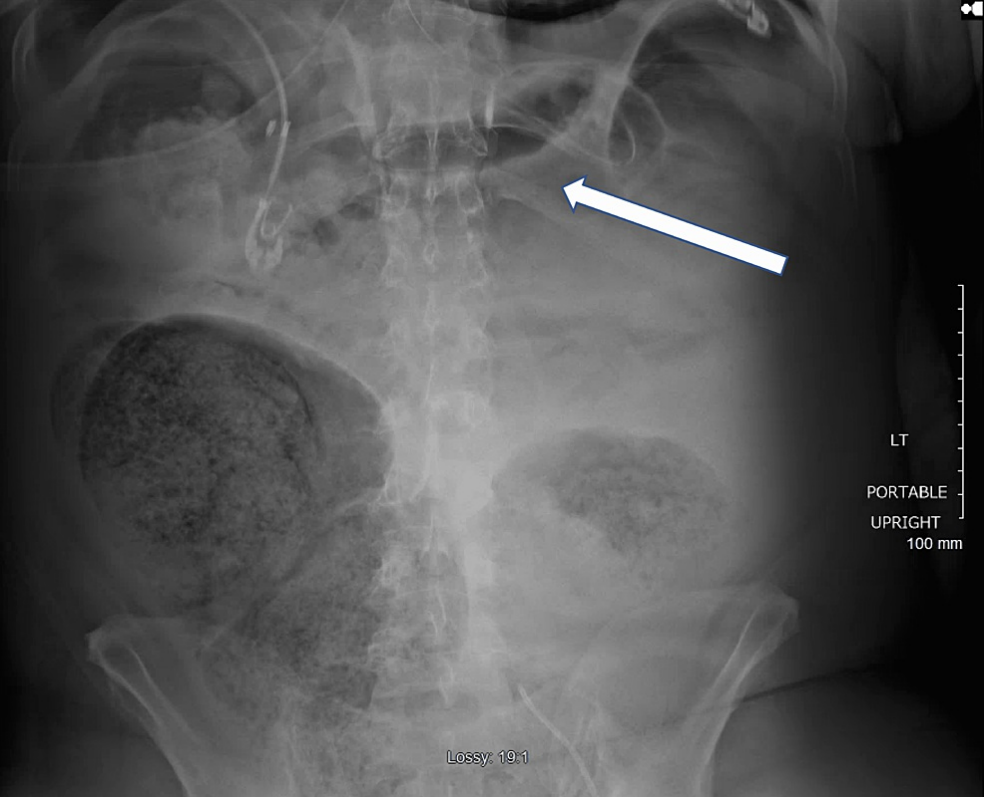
Plain upright abdominal plain film radiograph indicating free air under the diaphragm (white arrow).
